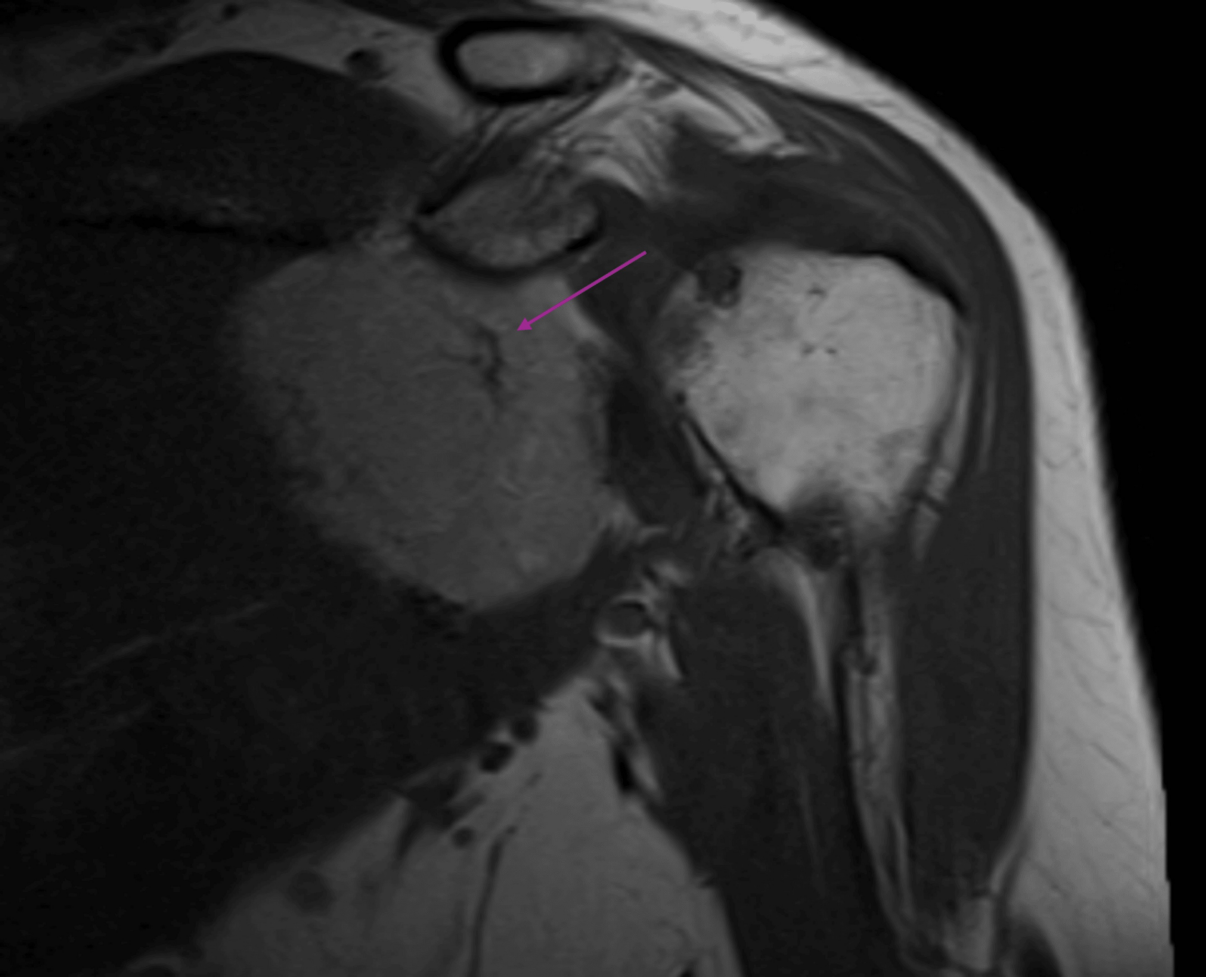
Oblique coronal T1-weighted MRI without fat suppression shows the presence of a mass, which is mildly hypointense to subcutaneous fat, with an intra-tumoral vessel within it (arrow).
